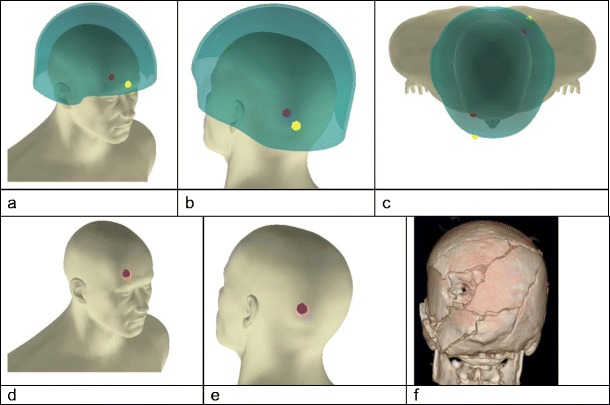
IMAP images from Incident 1. a Entry wounds, helmet in situ. b Exit wounds, helmet in situ. c View from above, helmet in situ. d Entry wound site on skin. e Exit wound site on skin. f Anonymised CT scan showing exit wound left parietal bone, posterior aspect
